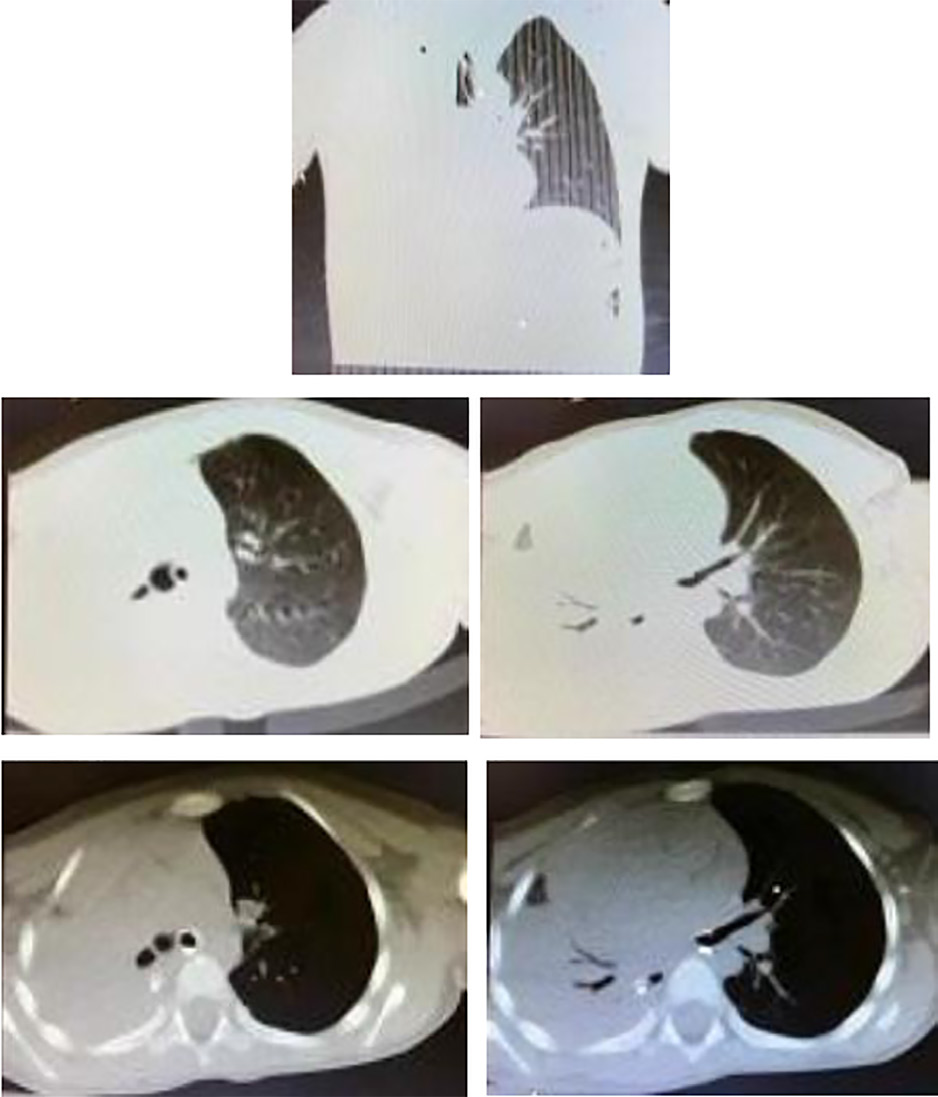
Lung Computed Tomography (CT) Scan of the patient
